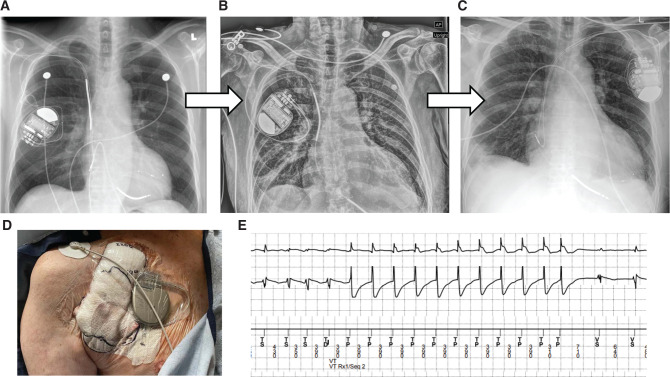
A: Admission chest X-ray showing the original right-sided cardiac resynchronization therapy (CRT-D) defibrillator dual-coil system. B and D: Temporary externalized single-chamber implantable cardioverter-defibrillator (ICD) system with a dual-coil DF1 RV ICD lead. C: Final left-sided CRT-D transvenous system following re-implantation 7 days after device extraction. E: Anti-tachycardia pacing therapy delivered by an externalized temporary ICD system successfully terminating ventricular tachycardia.
